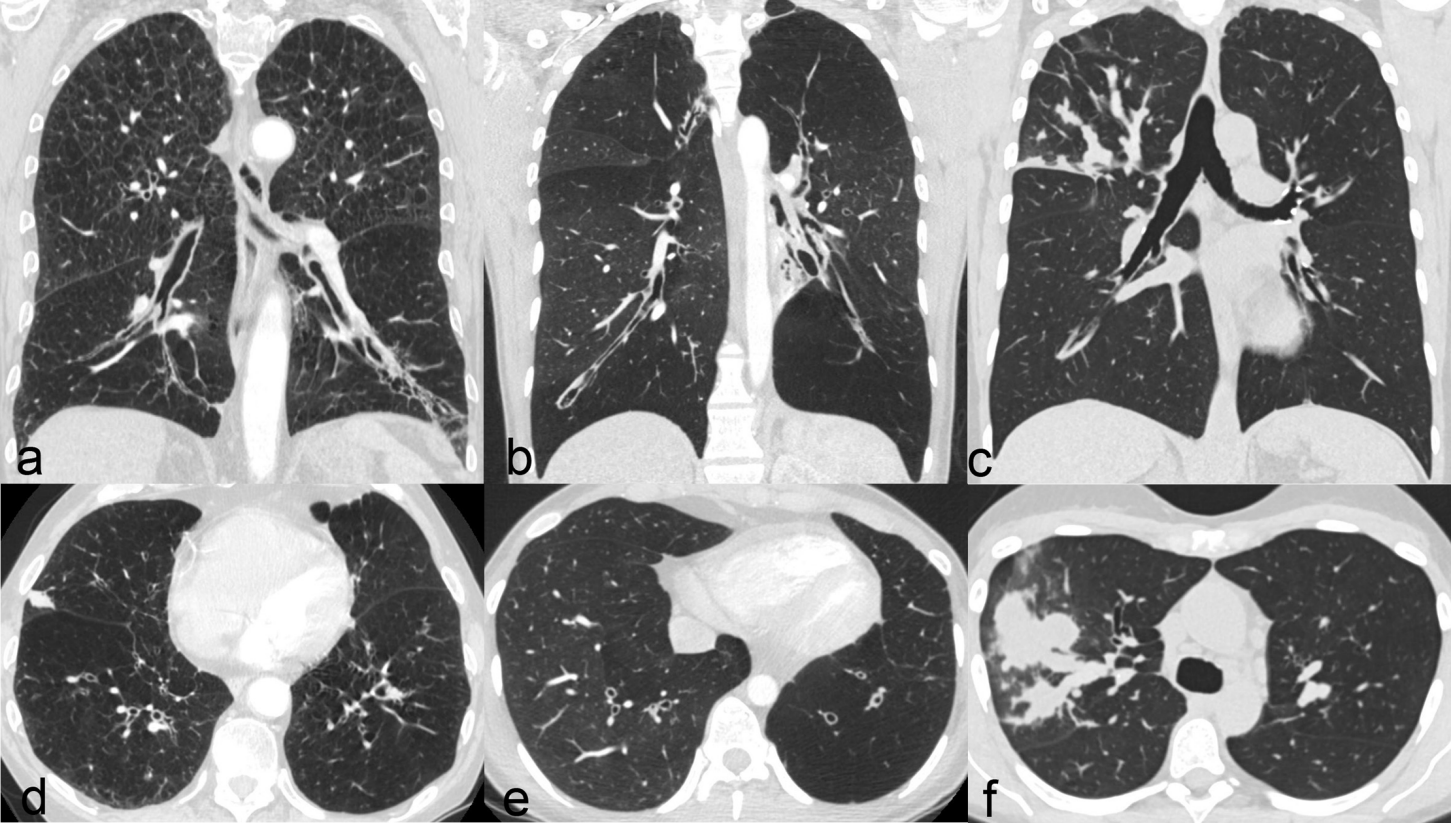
CT in patients with other underlying diseases. CT of three patients with bronchiectasis due to other underlying diseases. Patient 1 is a 74 year old male patient with COPD (a, d). Bronchiectasis are of cylindrical configuration and predominant in the lower lobes, collateral findings are the emphysematous changes. Patient 2 is a 20 year old woman with Swyer James Syndrome (b, e). There are severe bronchiectasis especially in the left lower lobe; the corresponding lung lobes are hyperinflated and the accompanying vessels are thin. Patient 3 (c, f) is a 53 year old female patient with allergic bronchopulmonary aspergillosis. There are focal varicose bronchiectasis in the right lower lobe with severe mucoid impactions.
